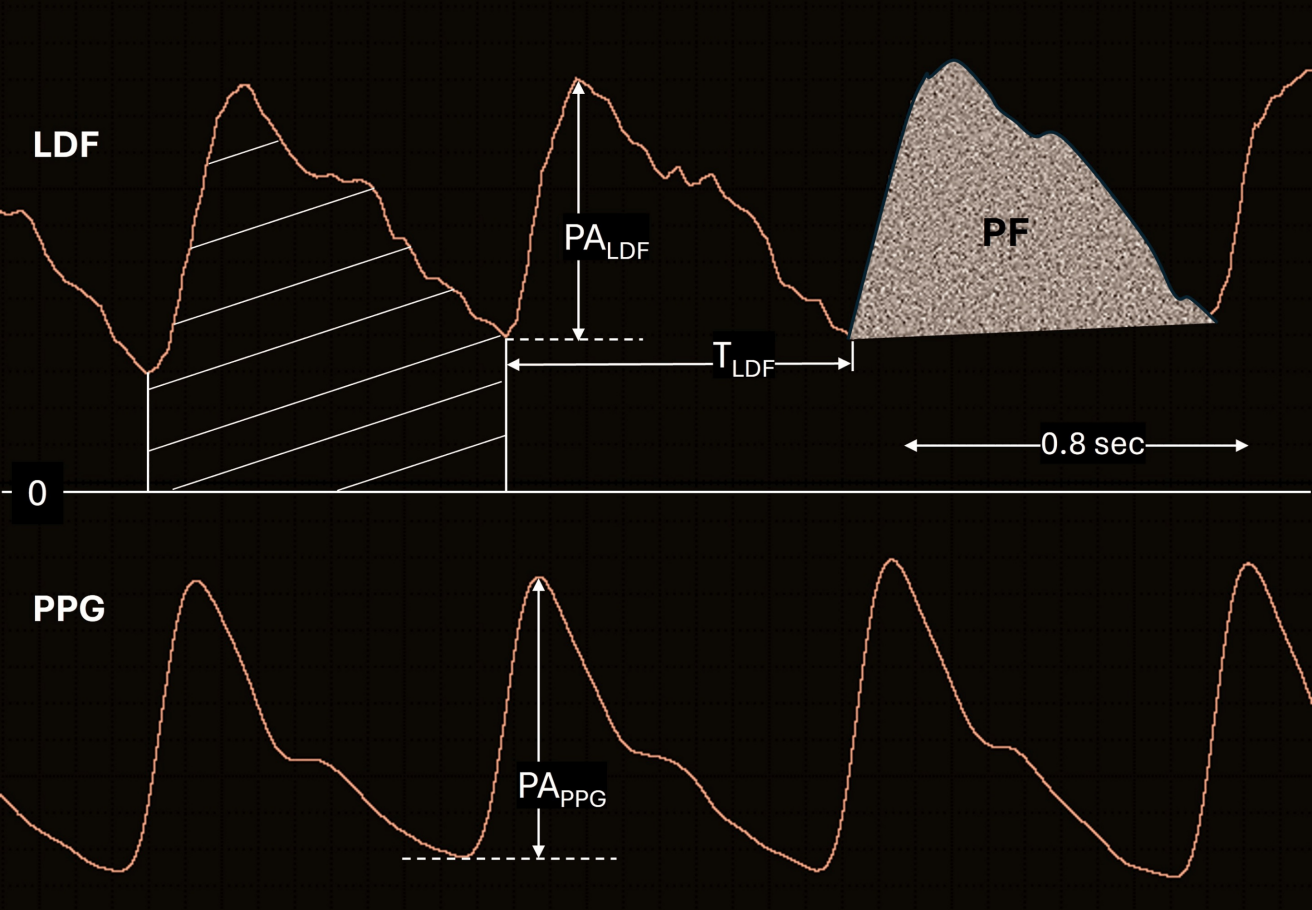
Parameter definitions LDF is the laser Doppler flux measured on the pulp of the ring finger. PPG is the photoplethysmography signal recorded from the index finger of the same hand.  Both are shown as a function of time. PALDF is the peak-to-peak amplitude of the LDF and PAPPG is the peak-to-peak amplitude of the PPG pulse. TLDF is the duration of the LDF pulse. The area with the diagonal lines in the LDF signal is the total LDF per pulse (LDFTOT). The area enclosed by the pulsatile part and labeled PF is the pulsatile flux component.
